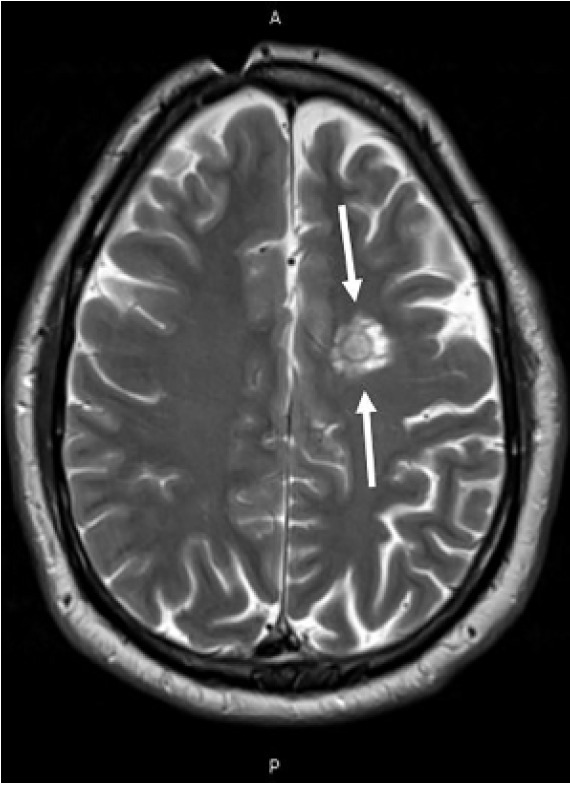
Magnetic resonance image of the head with contrast (T2 image) in a 50-year-old male with dizziness. White arrows identify a heterogeneous lesion in the left frontal centrum semiovale consistent with Balò’s concentric sclerosis.
